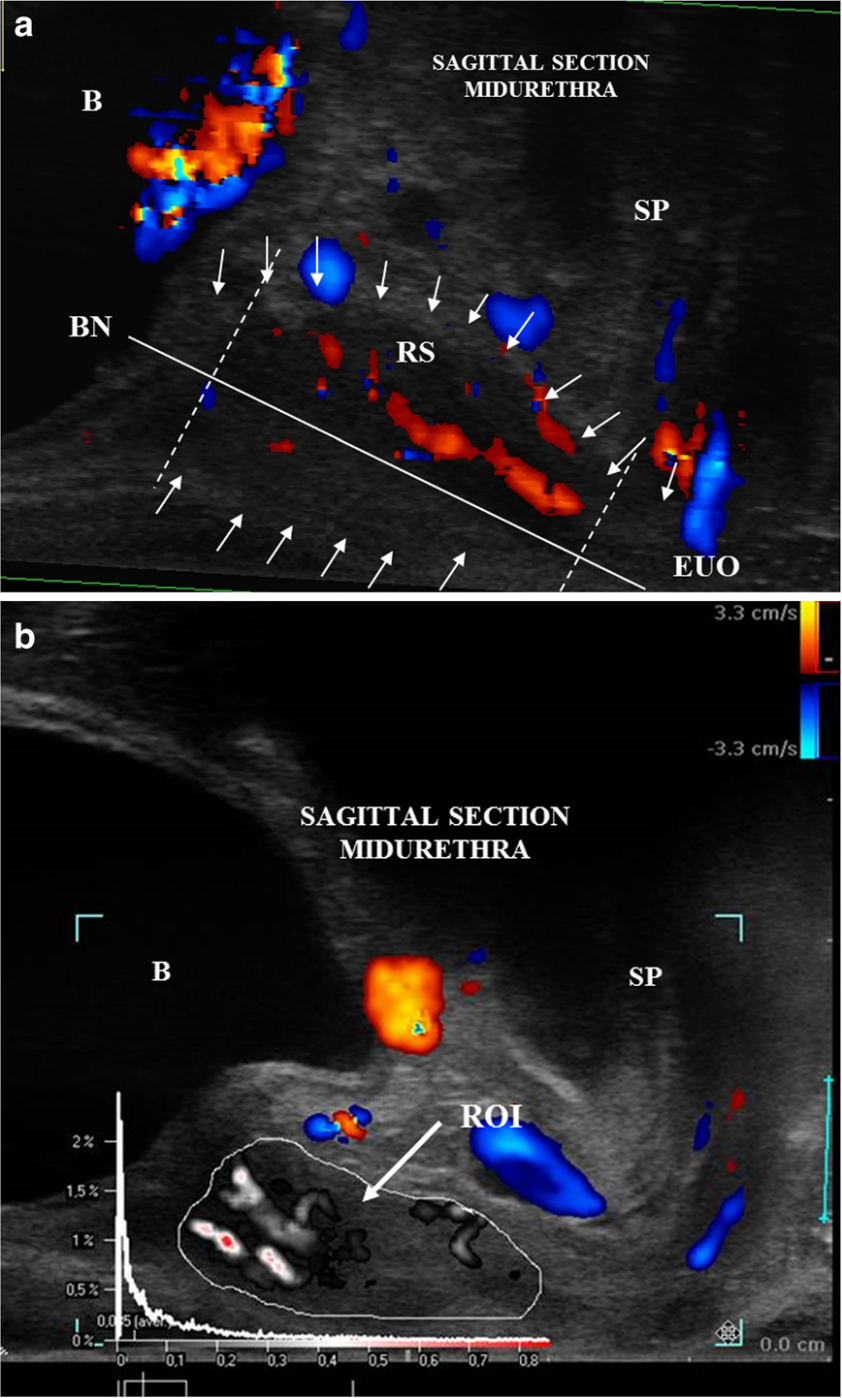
Endovaginal US and Pixel Flux technique in midsagittal section of the mid urethra. a Endovaginal US with the use of high-frequency 180 degrees rotational transducer (type 8848, B-K Medical) in midsagittal section of the urethra, colour Doppler mode. Arrows pointing the borders of the urethra. Area between the dotted lines demonstrates mid urethra. Straight line passing from bladder neck (BN) to external orifice of urethra (EUO) indicates urethral lumen. B- bladder, RS- rhabdosphincter, SP- symphysis pubis. b Pixel Flux technique: Region of interest (ROI) in midsagittal section of the urethra showing the local perfusion relief. B-bladder, SP- symphysis pubis.
